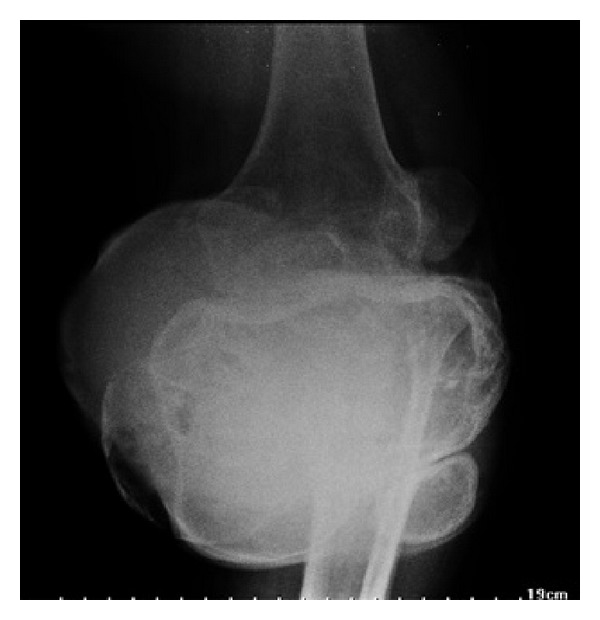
X-ray of the right knee showing osteolytic lesion in proximal tibia.
